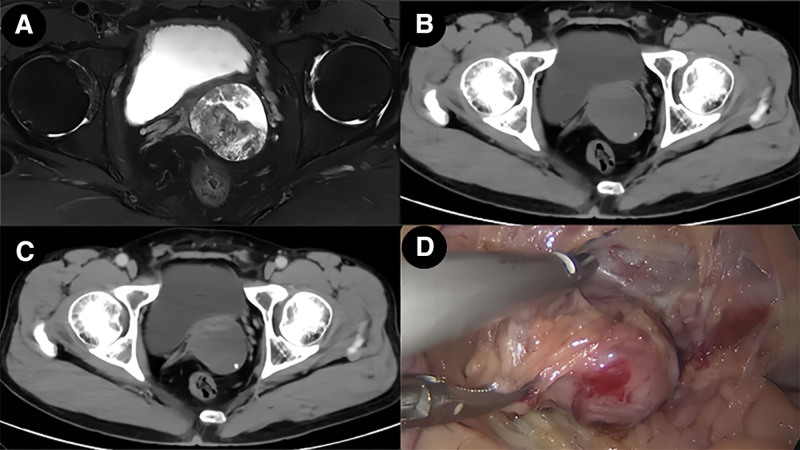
Patient’s pelvic MRI and CT plain scan + enhancement, intraoperative pictures. (A) MRI shows 2 mass-like abnormal signal foci between the left side of the pelvis, prostate, and left seminal vesicle, mostly presenting as long T1 and long T2 cystic. (B, C) CT plain scan + enhancement shows a mixed density mass in the left seminal vesicle area of the pelvis, with mild enhancement in the arterial phase and continued enhancement in the portal phase. (D) Intraoperatively, a round tumor about 5 cm in size was observed.
